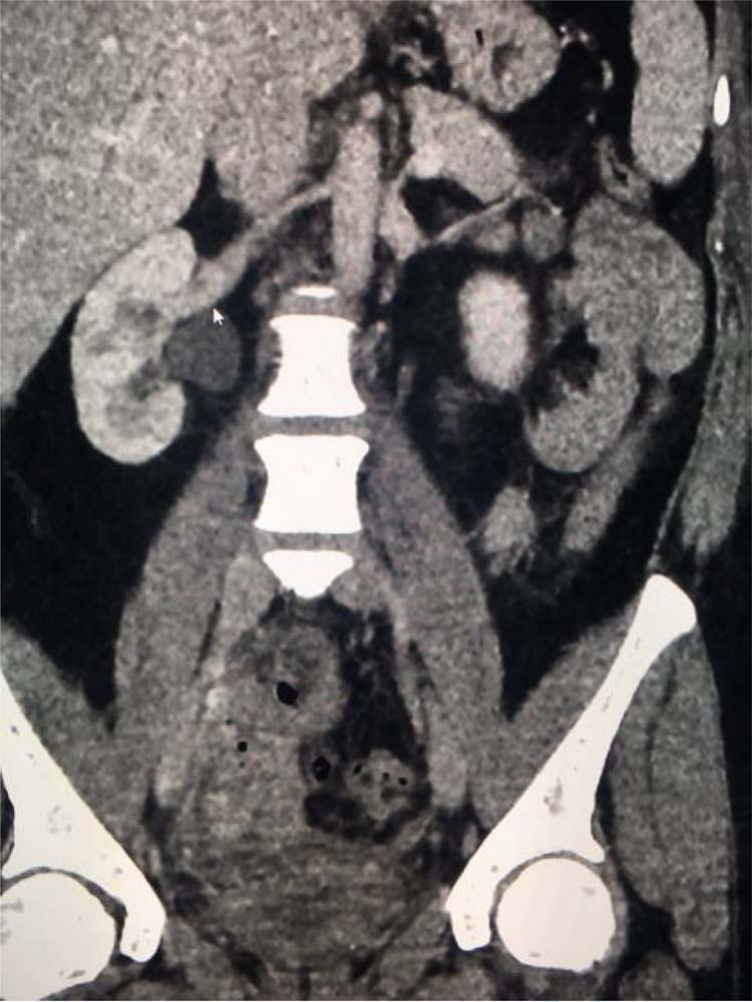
Coronal nephrogenic phase CT urogram showing hydronephrosis of the right renal pelvis.
